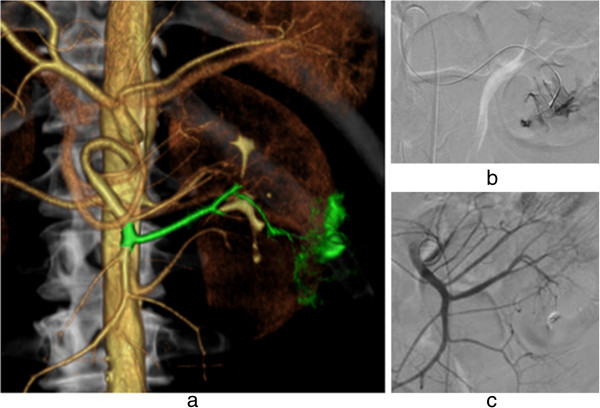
A case of endoscopically unidentified intestinal bleeding. (a) Hemorrhage-responsible arteriogram created by CT during abdominal aortography depicts the jejunal artery (green) as the source of the bleeding. Using this image, the jejunal branch artery was catheterized using a microcatheter (b), followed by embolization with n-butyl cyanoacrylate (c).
